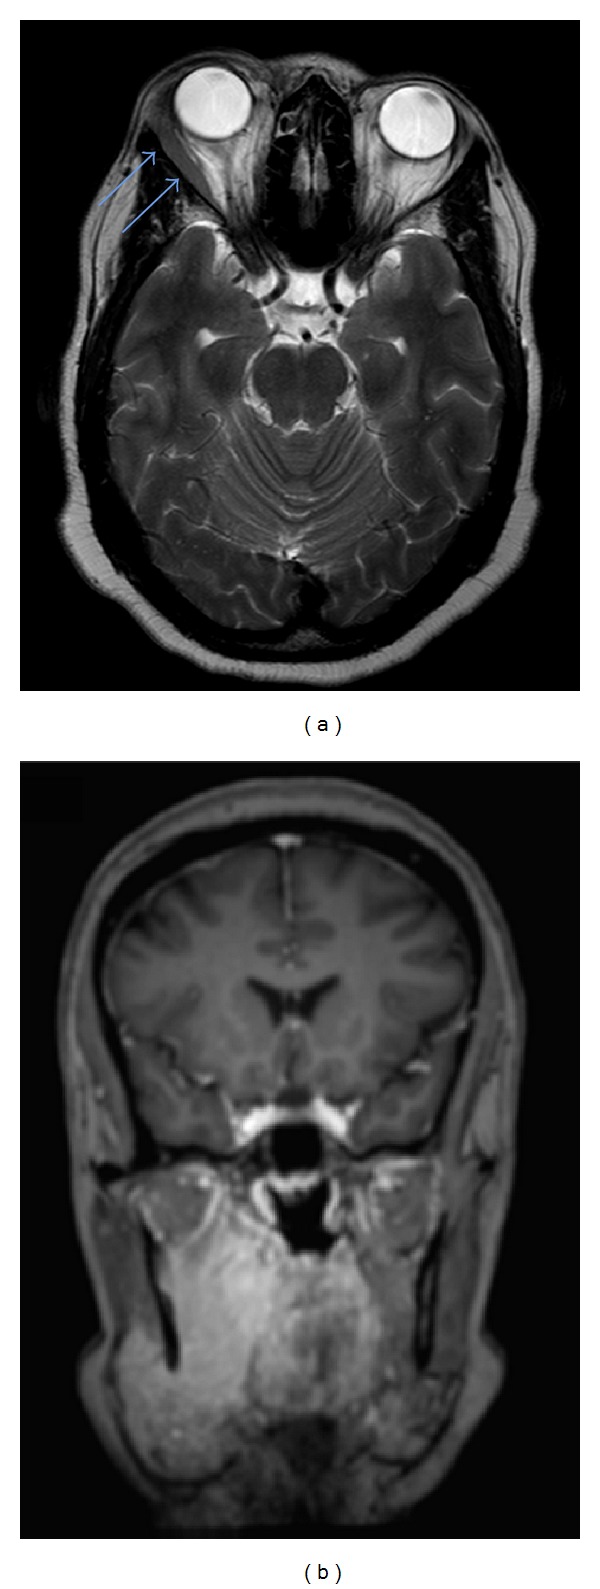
Head and neck magnetic resonance imaging*: axial T2 MRI revealed enlargement of the lateral rectus muscle of right orbit (arrows) (a). A coronal contrast-enhanced T1 MRI showed an infiltrating lesion centered in the right masticator space extending into the submandibular space involving the submandibular gland. Note the avid gadolinium enhancement pattern of the lesion and its ill-defined margins (b).
